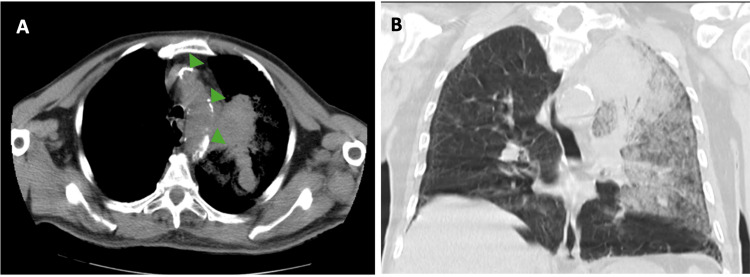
Chest CT angiography: (A) axial view showing calcification of the aorta (green arrowheads); (B) unenhanced coronal view, showing left pulmonary flooding in the context of aneurysmal rupture. CT, computed tomography
